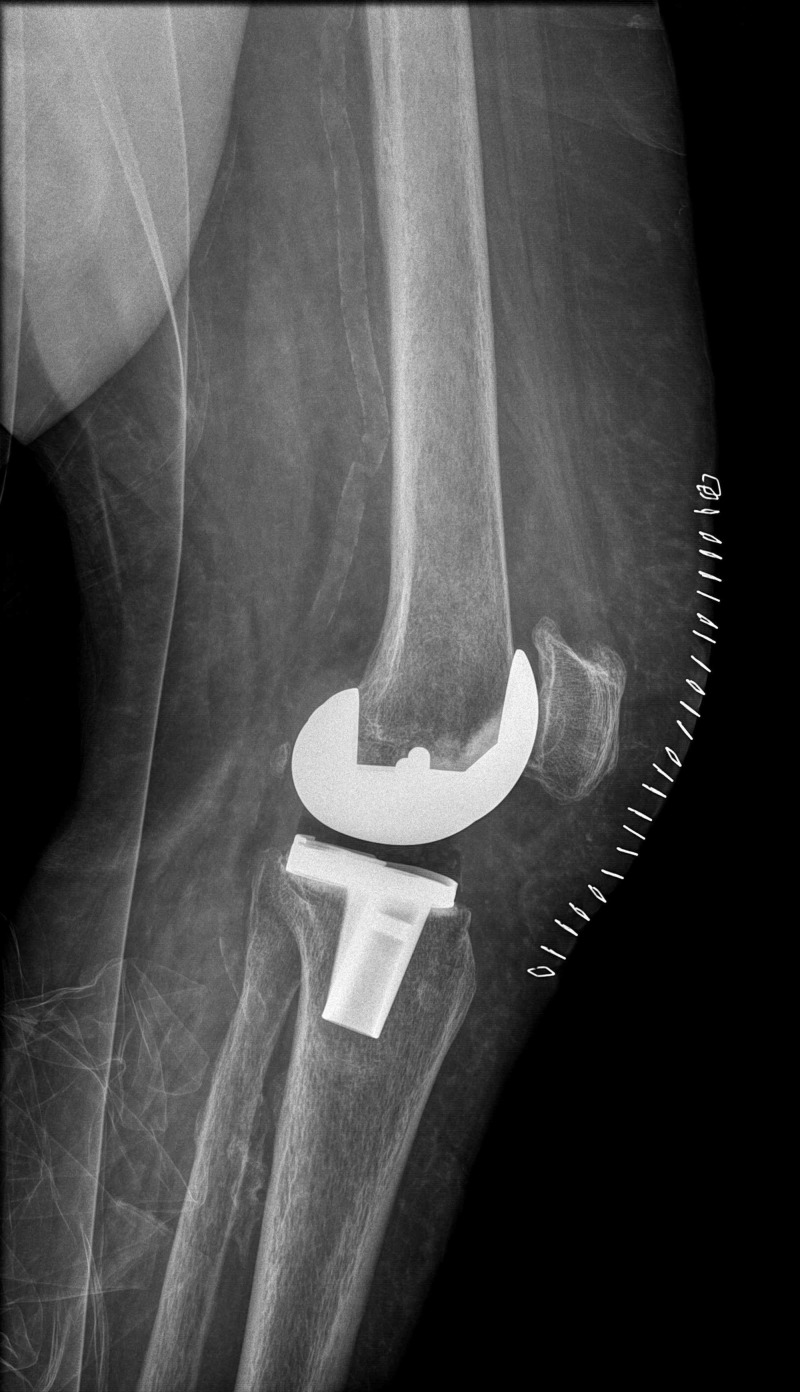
Immediate postoperative lateral left knee radiograph
